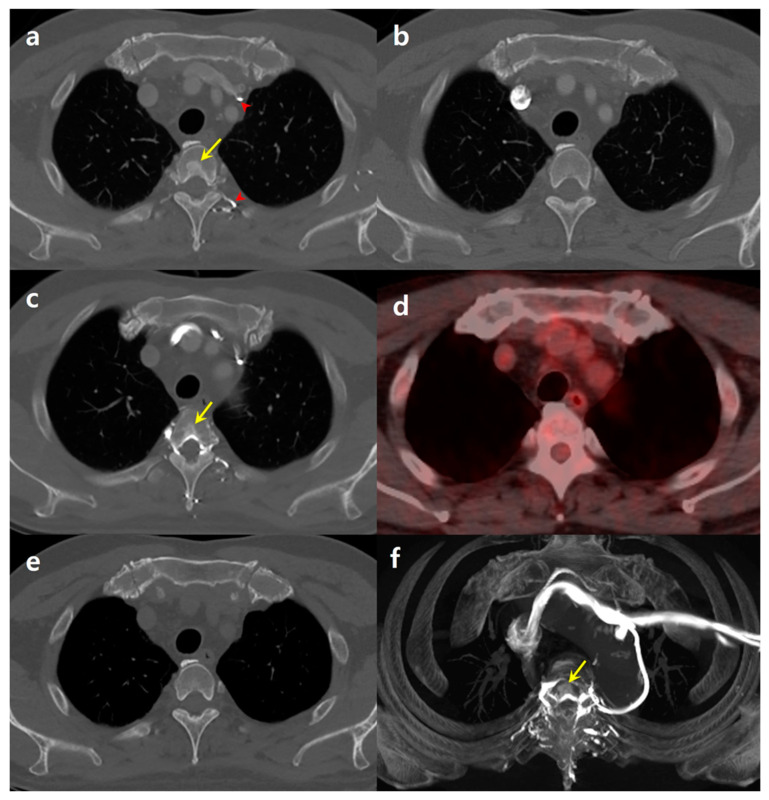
A 65-year-old male patient with a history of colon cancer surgery. (a) Contrast-enhanced chest computed tomography (CT) image, obtained with left-side contrast injection using bone window setting, reveals focal sclerotic area at the central portion of the T3 vertebral body (arrow). Notably, contrast arrival is observed in the left brachiocephalic vein and the left paravertebral venous plexus (arrowheads). (b) Chest CT obtained six months ago with right-side injection shows no evidence of a sclerotic lesion in the T3 vertebral body. (c) Chest CT obtained 11 months ago with left-side injection reveals the presence of focal sclerotic lesion (arrow) in the same location. (d) 18F-FDG PET-CT reveals no abnormalities in the same vertebra site. (e) A follow-up unenhanced chest CT conducted six months later from (a) shows no sclerotic lesion. (f) Maximum-intensity projection (MIP) image reconstructed from an enhanced CT reveals a focal enhancing area at T3 (arrow), with the surrounding vertebral venous plexus opacified through reflux from the left brachiocephalic vein.
